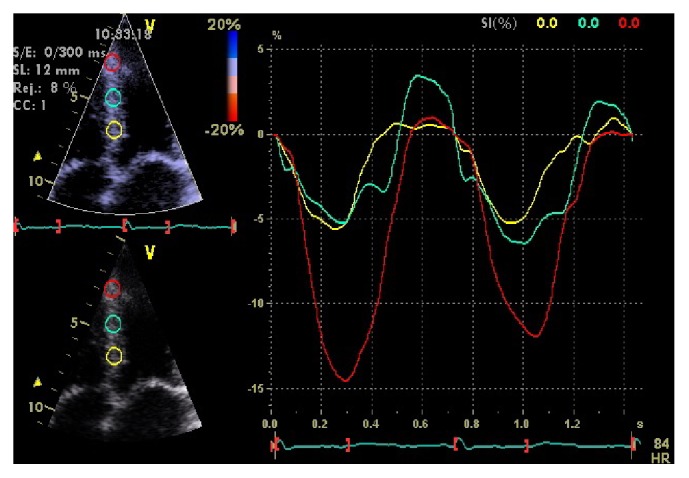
Strain curves from the interventricular septum of the same patient appearing in Figures 2 and 3. In this example, strain curves were constructed with the Doppler Myocardial Imaging technique. There is reduced peak systolic strain of the basal (yellow curve) and mid (green curve) septal segments, compared to the apical segment (red curve) with an apex to base ratio > 2.1.
